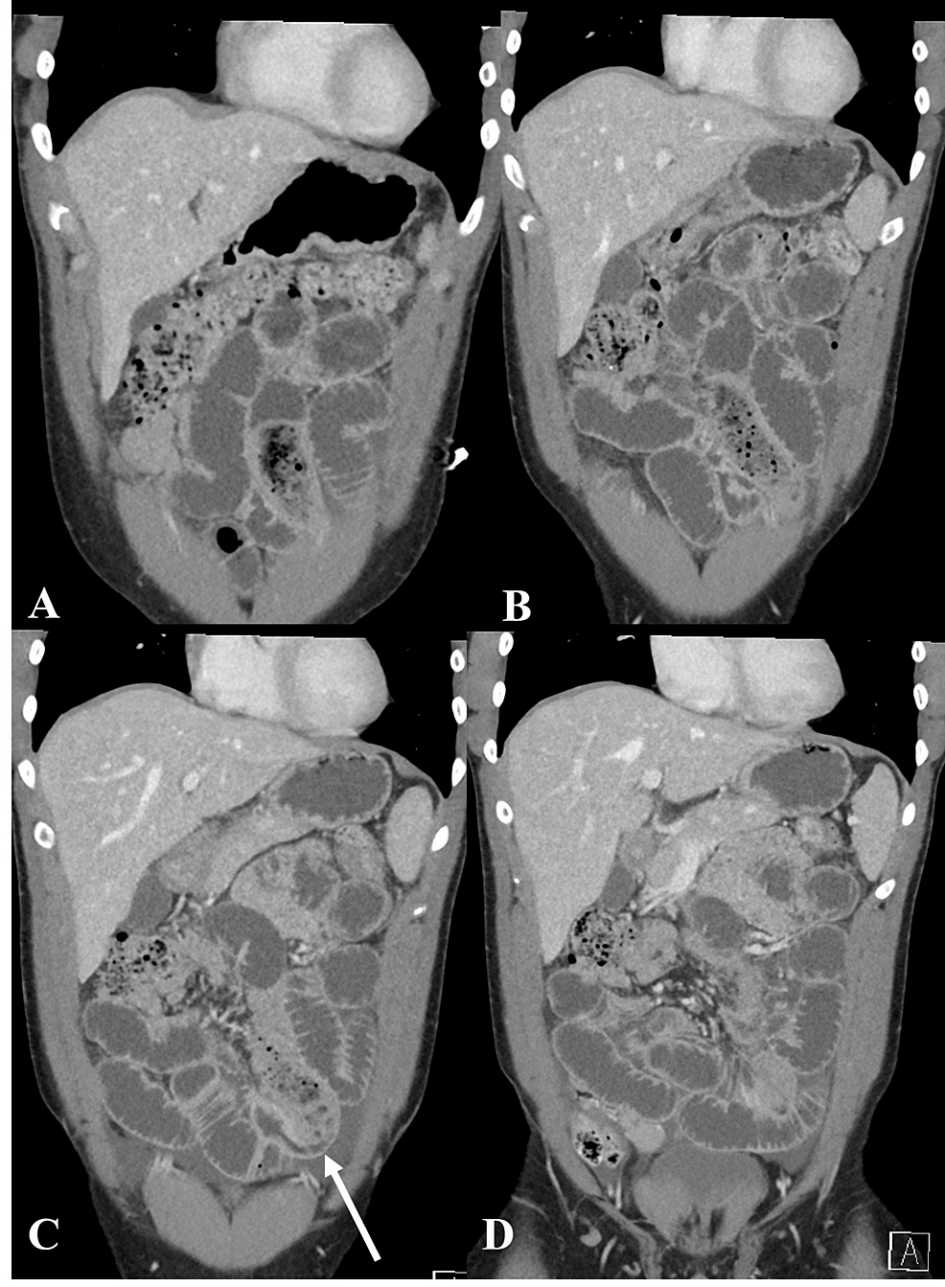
Dilated small bowel loops and transition point of the obstruction Contrast-enhanced CT of the abdomen and pelvis in coronal reformation from anterior to posterior (A-D) shows multiple dilated small bowel loops with fecalization of the contents in the left lower quadrant, and a transition point (white arrow).
